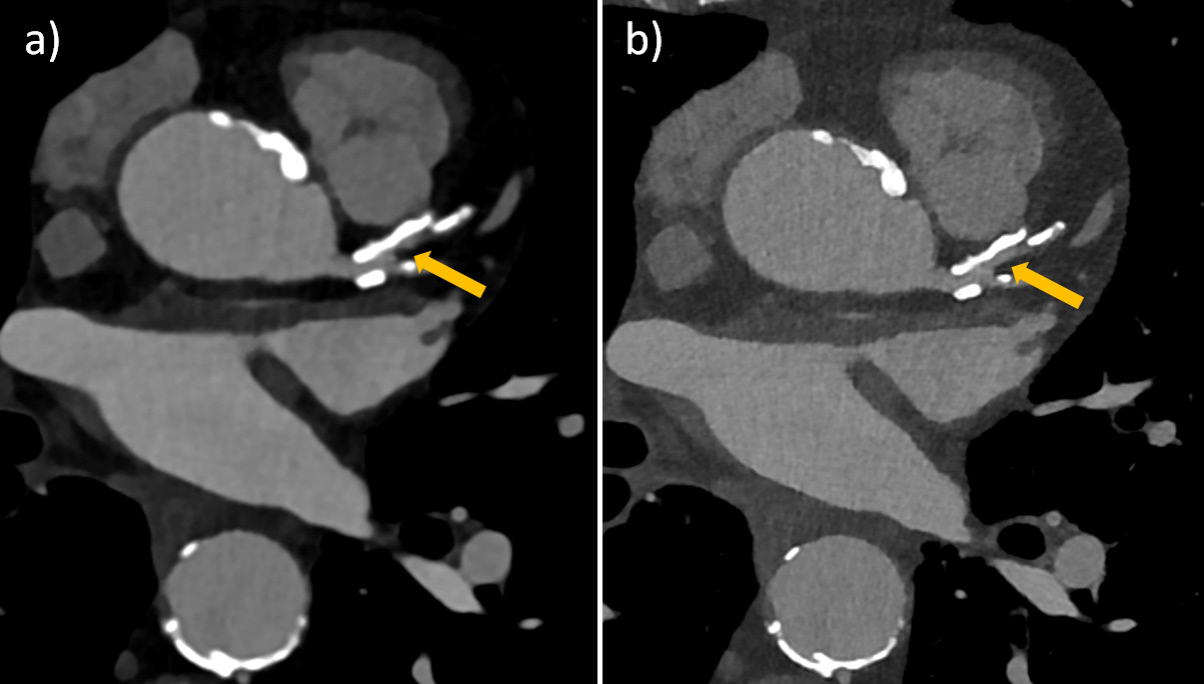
Ultra-high-resolution cCTA of an 82-year-old male patient on a clinical PCD-CT. Heart rate during data acquisition: 66 bpm. (a) Standard reference reconstruction (0.6 mm slice, Bv40, QIR4). (b) UHR reconstruction (0.2 mm slice, Bv56, QIR4). Note the improved visualization and larger perfused diameter (arrow) of the proximal LAD in the UHR image, which appears almost occluded in the standard reconstruction. cCTA, coronary CT angiography; PCD-CT, photon-counting detector CT.
